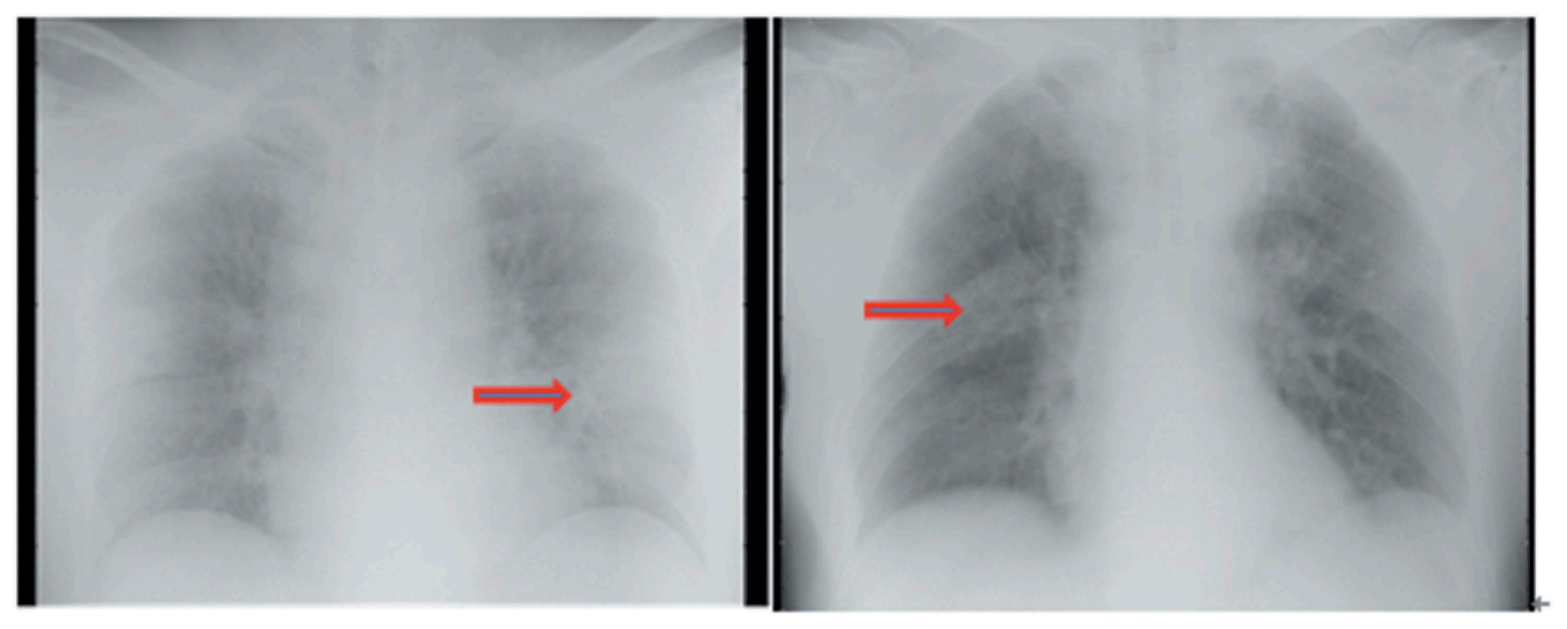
Typical change on chest radiographs of PT 10. The brightness of both lungs was decreased. Multiple patchy shadows with blurred edges were observed.
